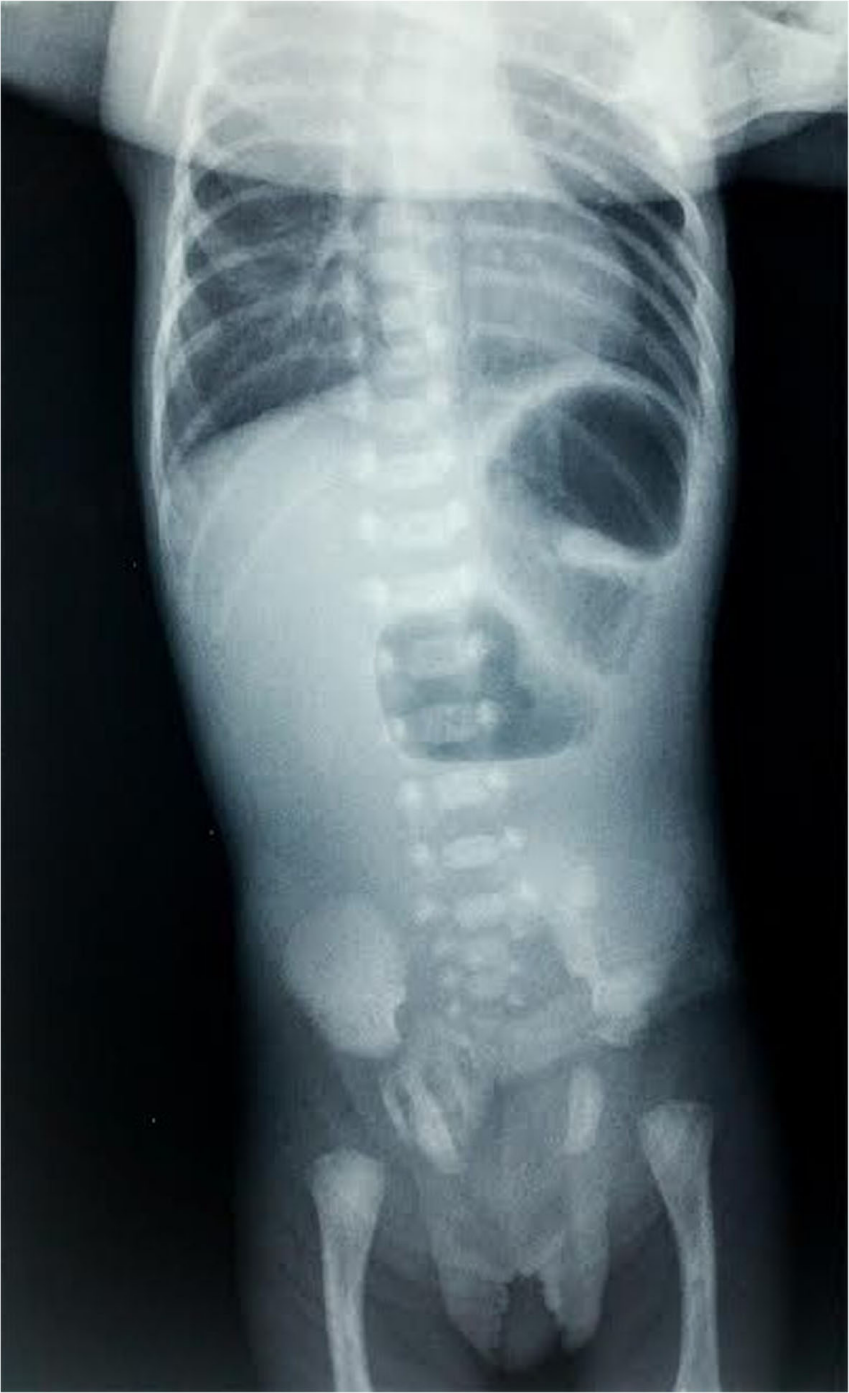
X-ray flat plate abdomen-showing clasical double-bubble apperance of duodenal atresia
